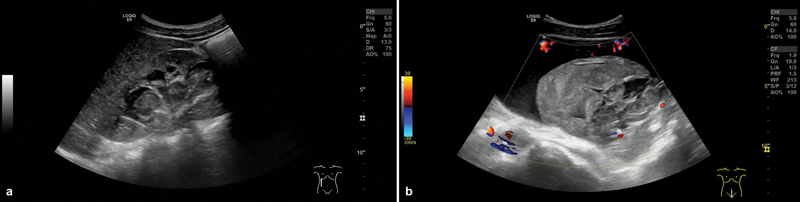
Ultrasound during the initial assessment for further clarification of the hematuria. (
